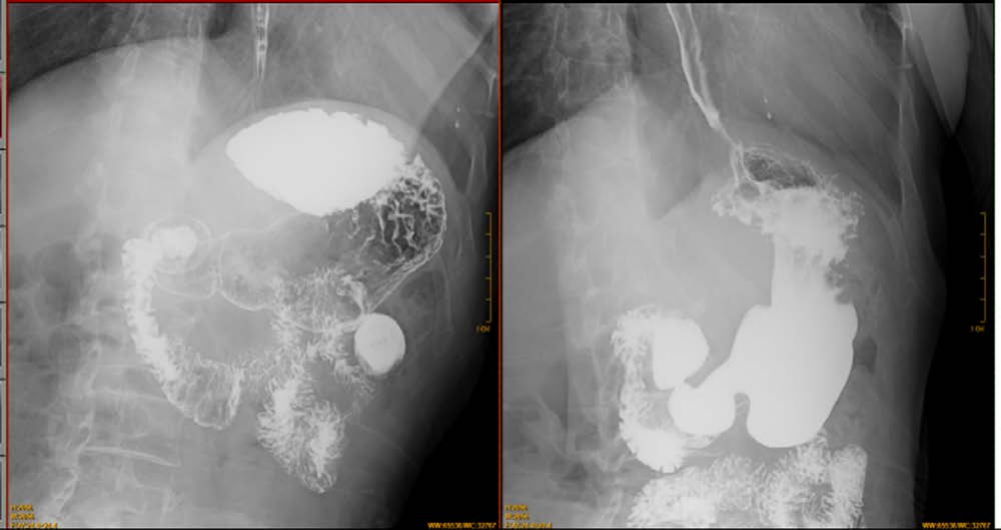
The digital gastrointestinal radiography (GI) showed a gastric carcinoma occurred in stomachus cardiacus and gastric body.
